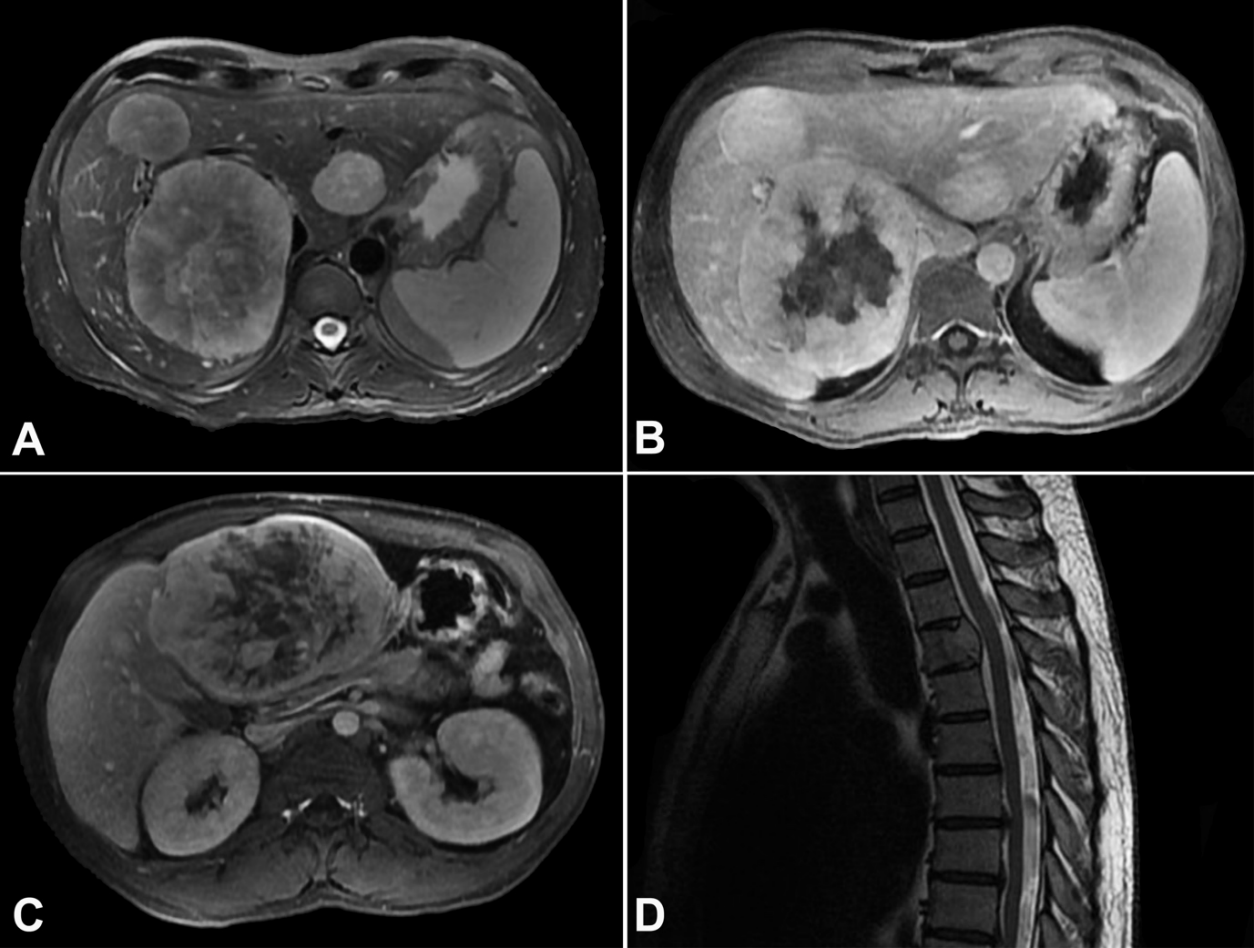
MRI scans in axial planes show multiple hepatic nodules distributed over both lobes. A – (T2-weighted sequence), the largest hypervascularized with extensive central necrosis, measuring 11.2 x 8.8 cm in segment VII/VII; B – (DCE sequence) and in segment III, measuring 12.5 x 9.3 cm; C – (DCE portal sequence); D – osteolytic lesions in the vertebral body of D4 (T2-weighted sequence in the sagittal plane), with a soft tissue component determining compression on the dural sac.
